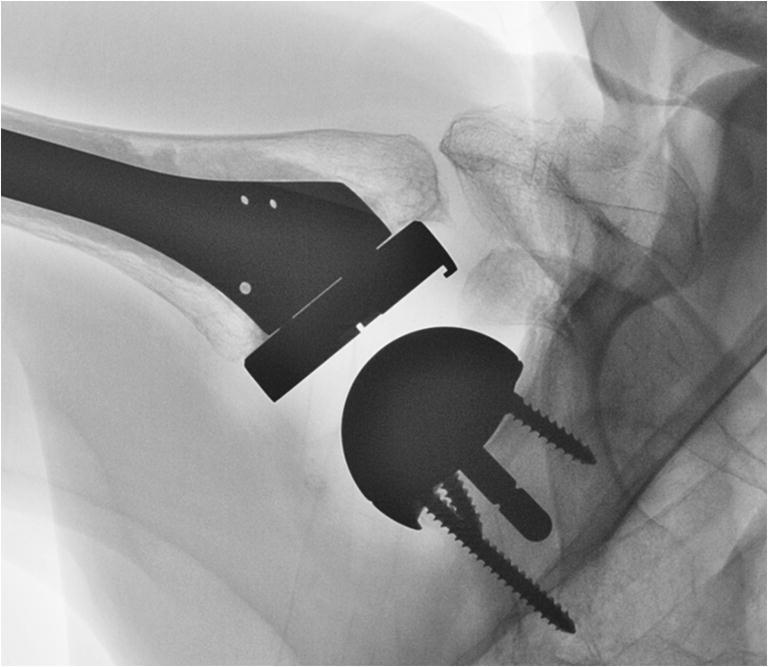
Anteroposterior radiograph of a Duocentric reverse prosthesis demonstrates a mechanical conflict between the greater tuberosity and the non-fractured acromion during abduction in the scapular plane
